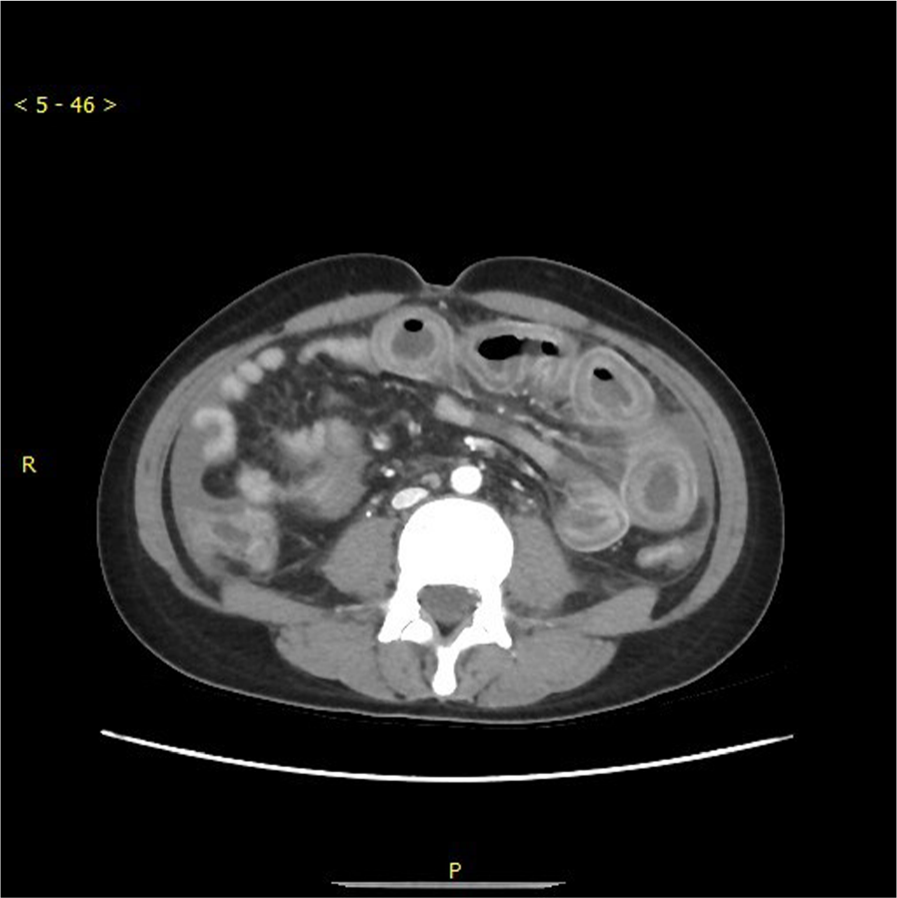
Transverse section CT scan showing dilatation of small intestine and ascites
